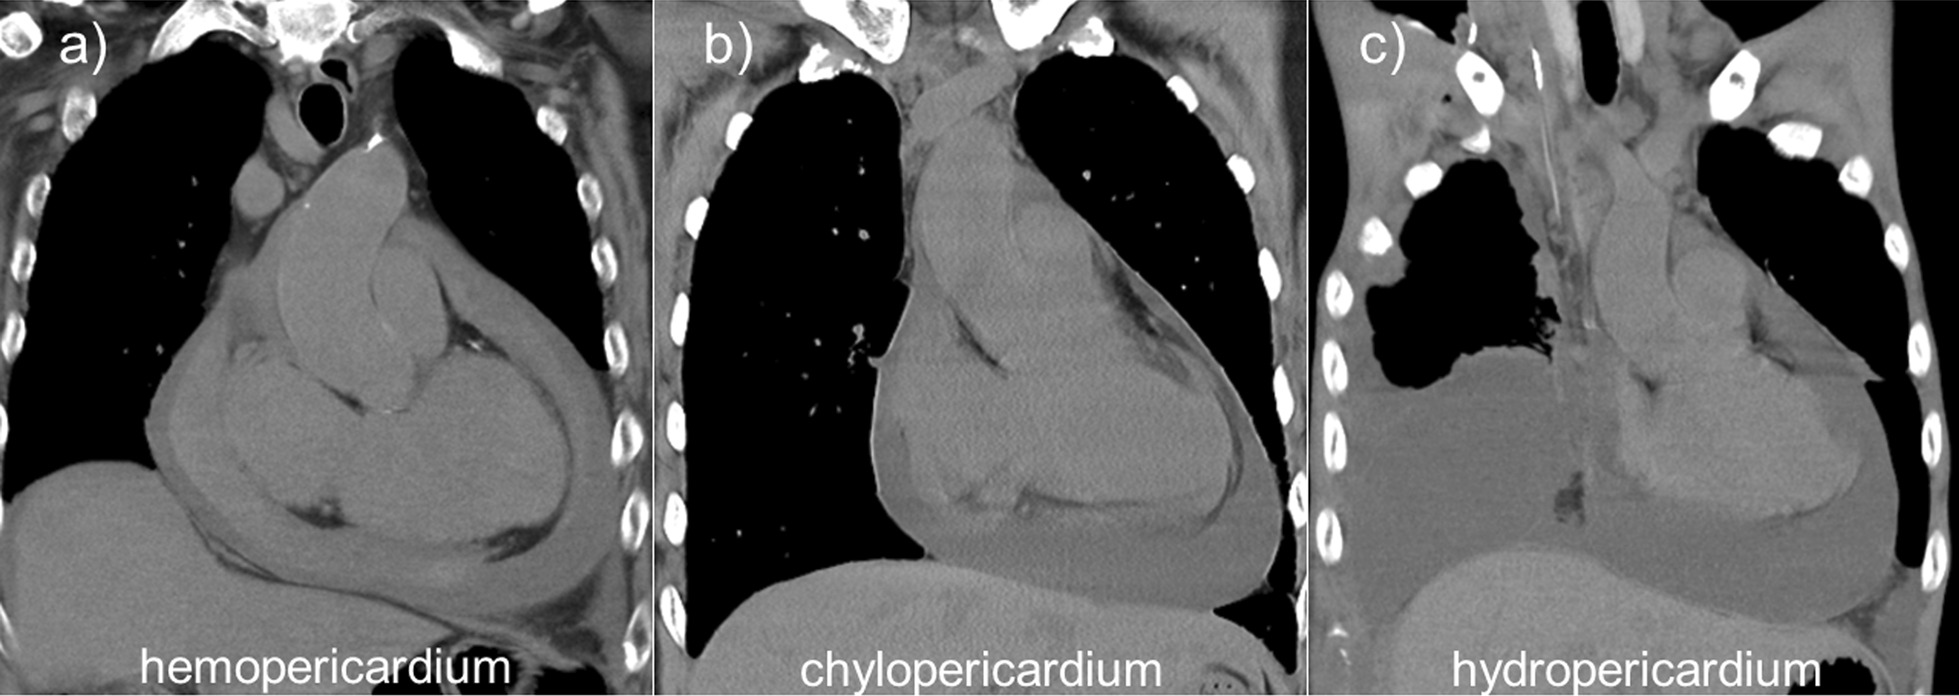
Computed tomography (CT) images comparing a hemopericardium, b chylopericardium, and c hydropericardium. The mean Hounsfield units (HU) on the CT scan of hemopericardium (22 cases) and hydropericardium (15 cases) were 22.32 and 15.39 HU, respectively. For chylopericardium (1 case, presented in the current report), it was 11.00 HU
